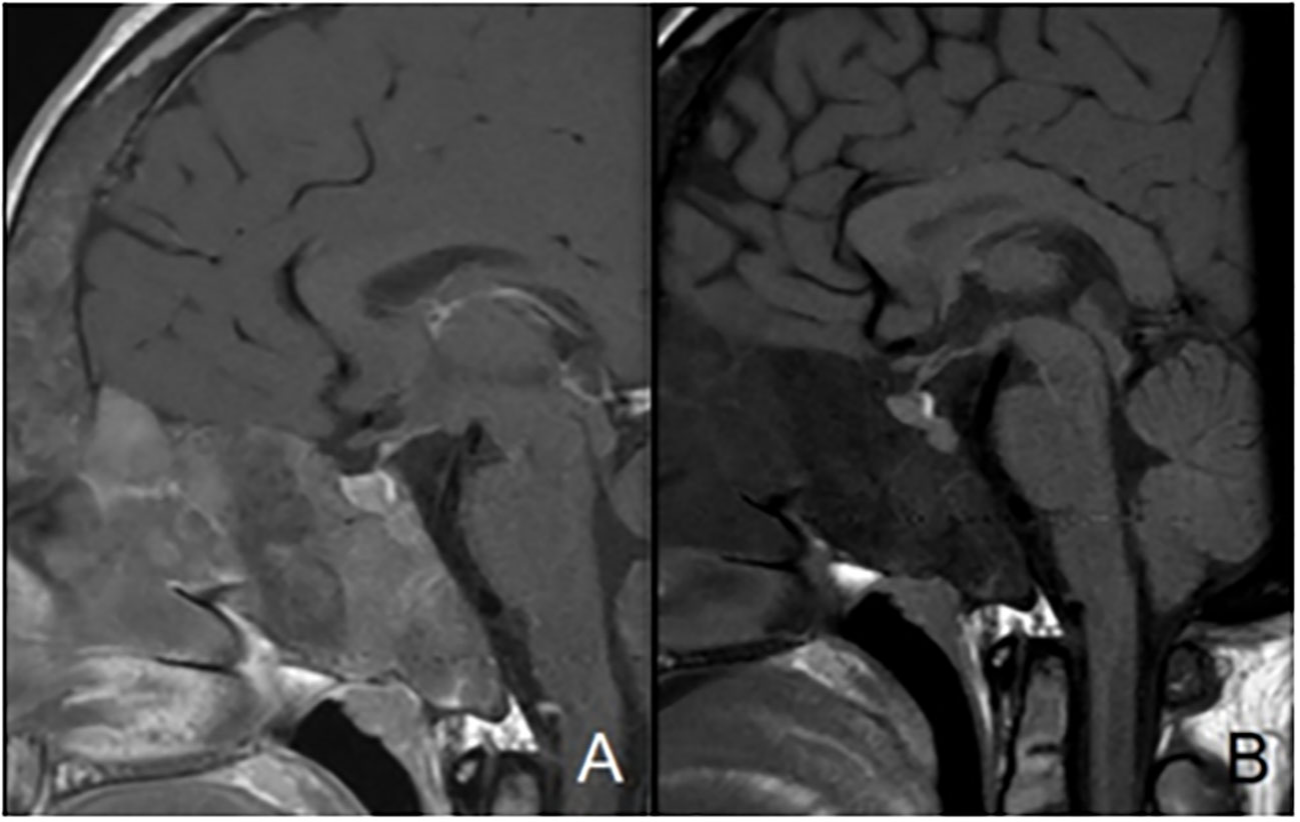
Mid-sagittal T1-weighted images with (A) and without (B) contrast-enhancement showing normal posterior pituitary gland and pituitary stalk and increased anterior pituitary hyperplasia in a patient with sphenoidal and clival bone dysplasia.
